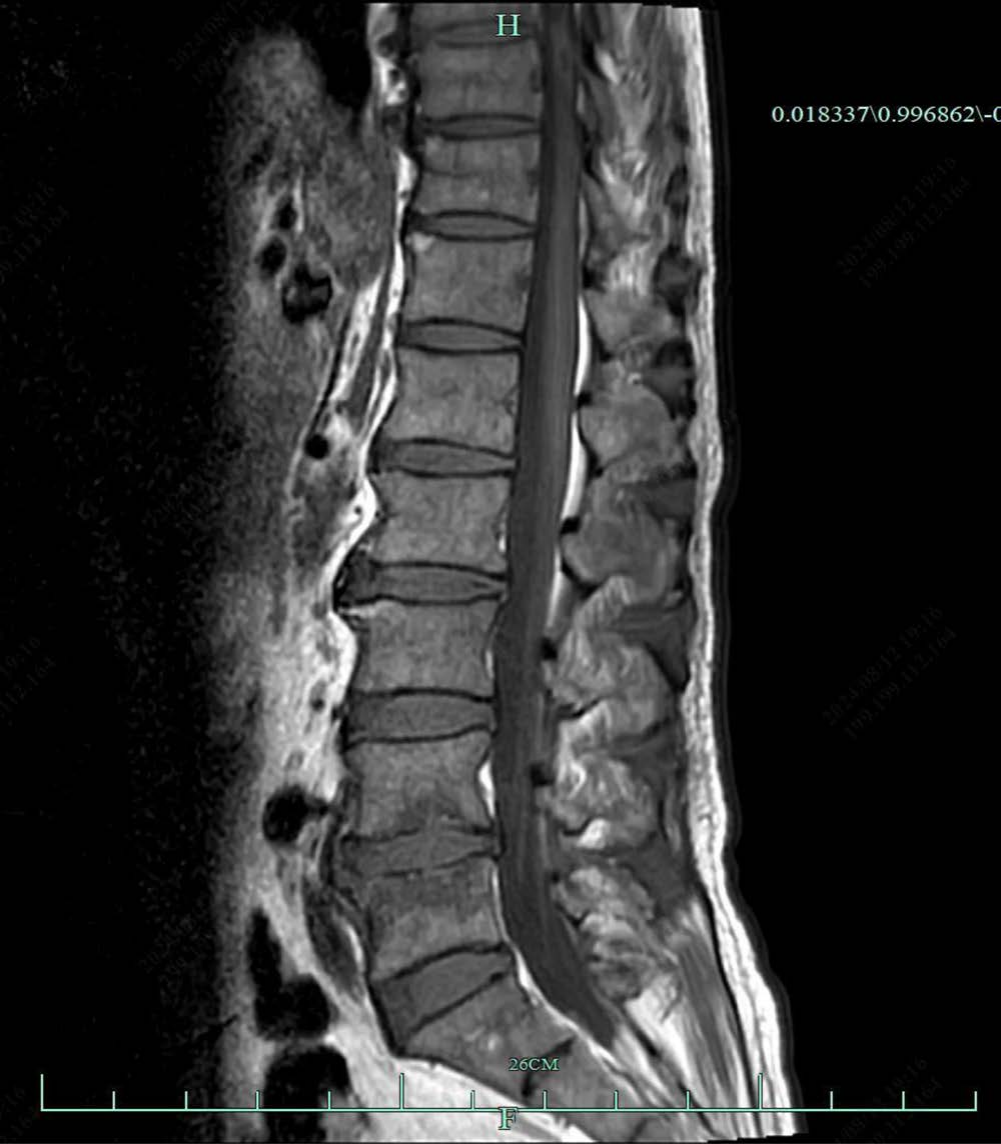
Lumbar MRI shows endplate inflammation at the lumbar vertebrae and exudative changes around the spinous processes and muscle spaces in the lower back. MRI = magnetic resonance imaging.
